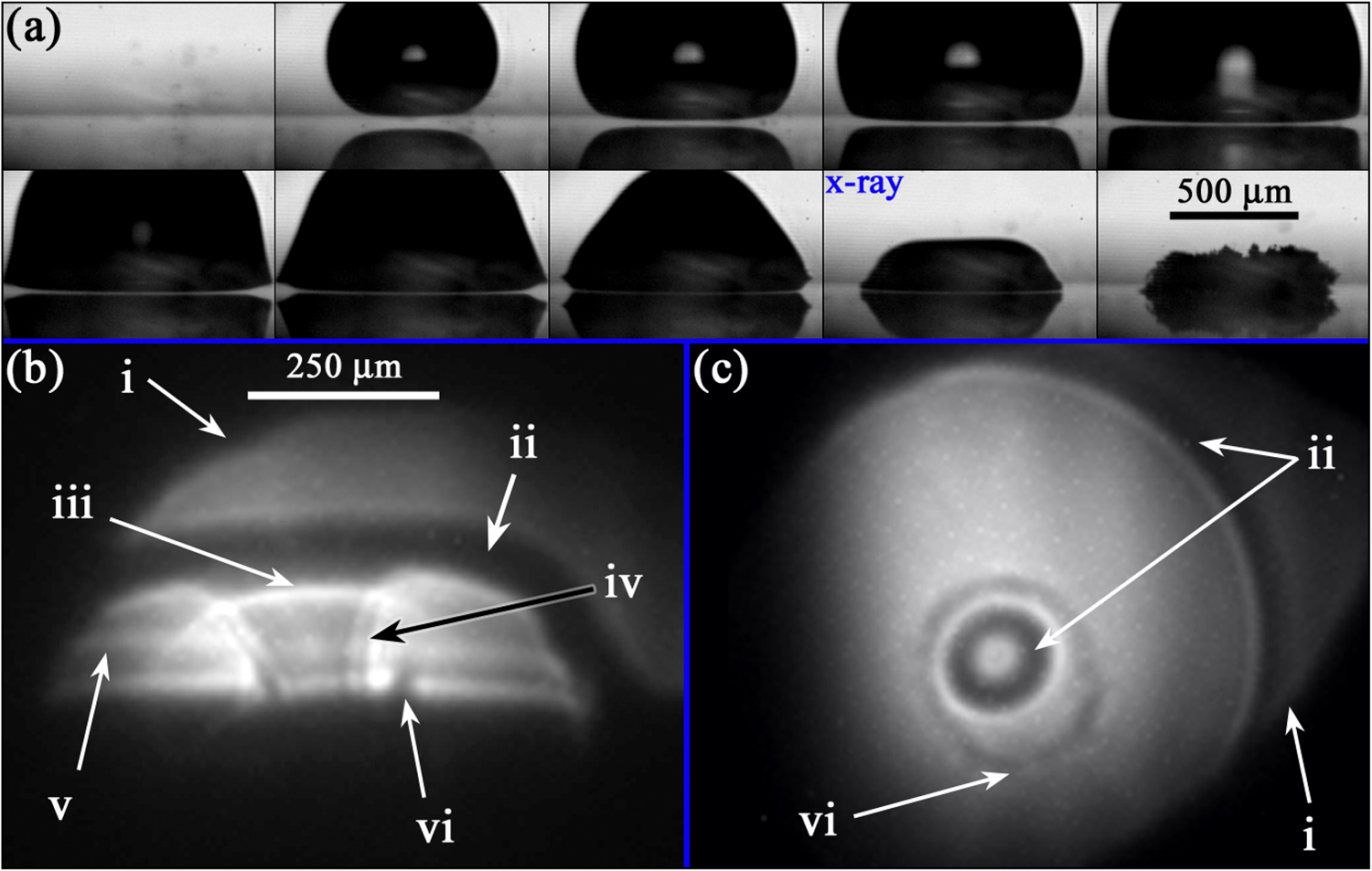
Optical and x-ray holographic imaging of a bubble jetting towards a rigid (glass) surface. a Optical image sequence of the bubble dynamics taken with 75 kfps (i.e. $$13.3\,\upmu {s}$$ between frames). Here, the stand-off distance is $$\gamma =0.57\pm 0.03$$. The lower panels present x-ray holographic images captured at the corresponding instant marked with the legend “x-ray” in (a). Panel b shows a side view of the jetting cavity as an X-ray hologram, while in (c) the jetting of a second bubble with similar dynamics is observed from a parallel view (normal to the glass disc). The x-ray light reveals the bubble interior, invisible in the optical shadowgraphs of (a). The arrows indicate some of the features commonly present in the holograms: i x-ray light blocked by the border of the hole of the laser mirror; ii thick interfacial line; iii bright arc; iv double shadow at the jet liquid column; v bubble pedestal stripes; and vi Blake splash
